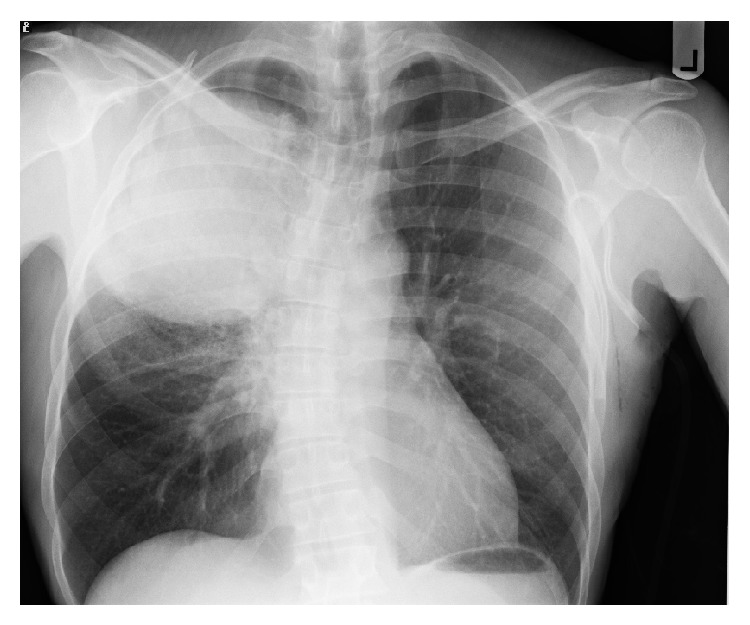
Chest X-ray: Rt upper lobe mass.
